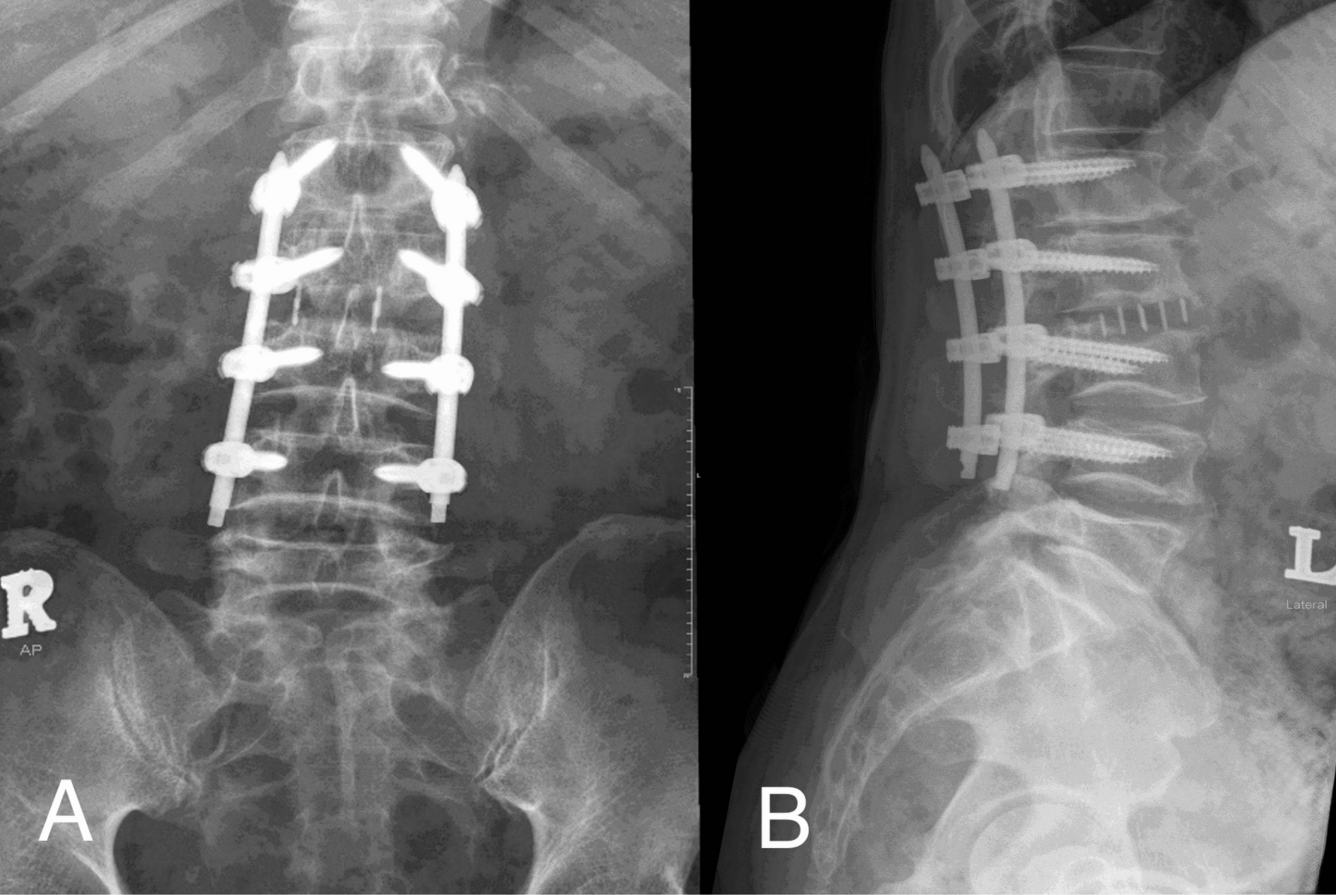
Postoperative lumbo-sacral X-ray to evaluate the placement of implant and fusion (A) Antero-posterior view showing the cage placement which included bi-cortical extension of the vertebral body. The alignment was restored and added further stability to the construct (B) Lateral view to evaluate the restoration of sagittal balance and the assessment of fusion at the level of cage placement
